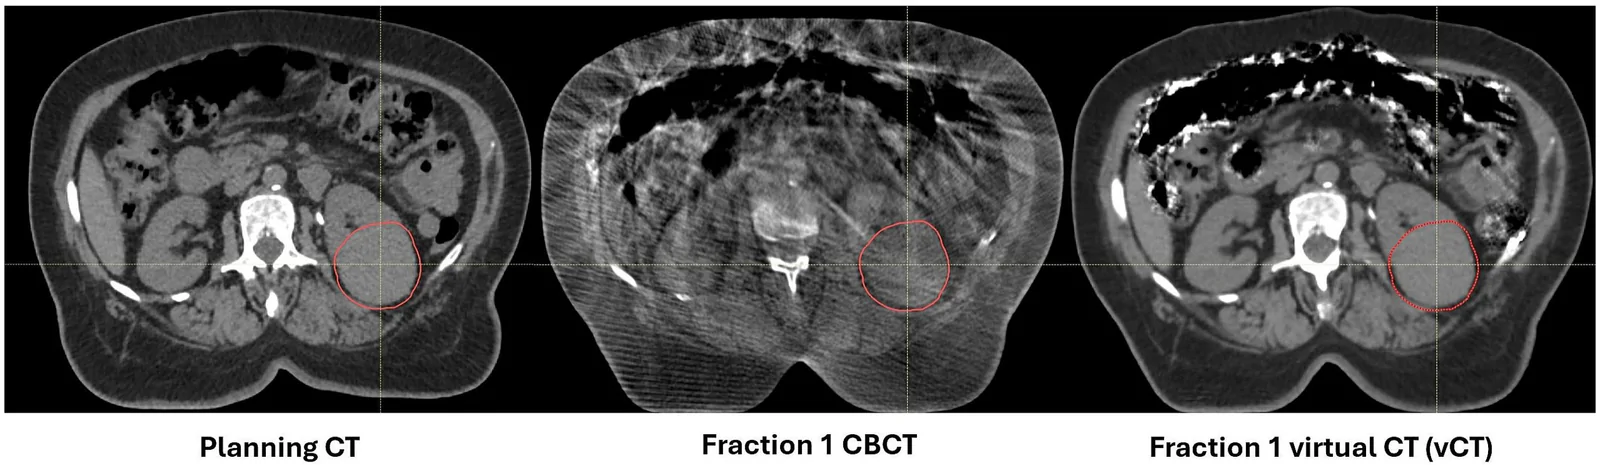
Sample virtual CT generation. The HU values were mapped from the treatment planning CT scan to the anatomy in the day-of-treatment CBCT scan, generating the virtual CT (vCT). The target (iCTV) is delineated in red. (For interpretation of the references to colour in this figure legend, the reader is referred to the web version of this article.)
